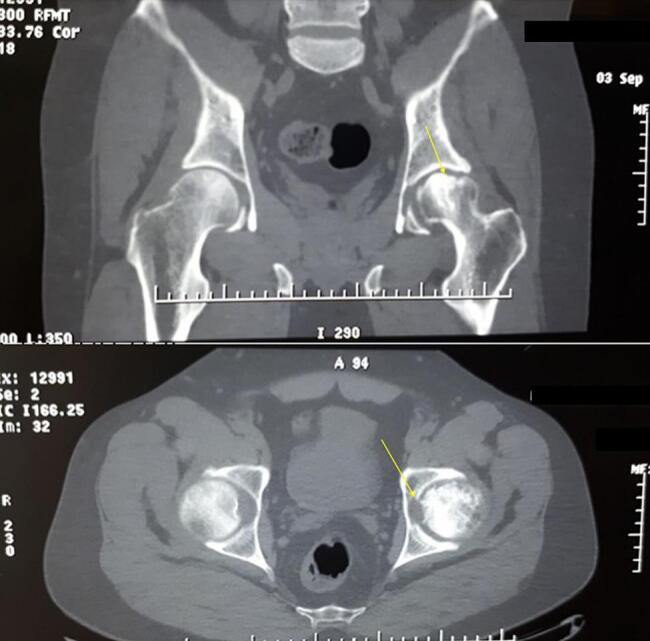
Axial (A) and coronal (B) view of pelvic CT scan. Left hip involved with femoral head collapse, subchondral hyper-density, osteoporosis, and osteonecrosis.
